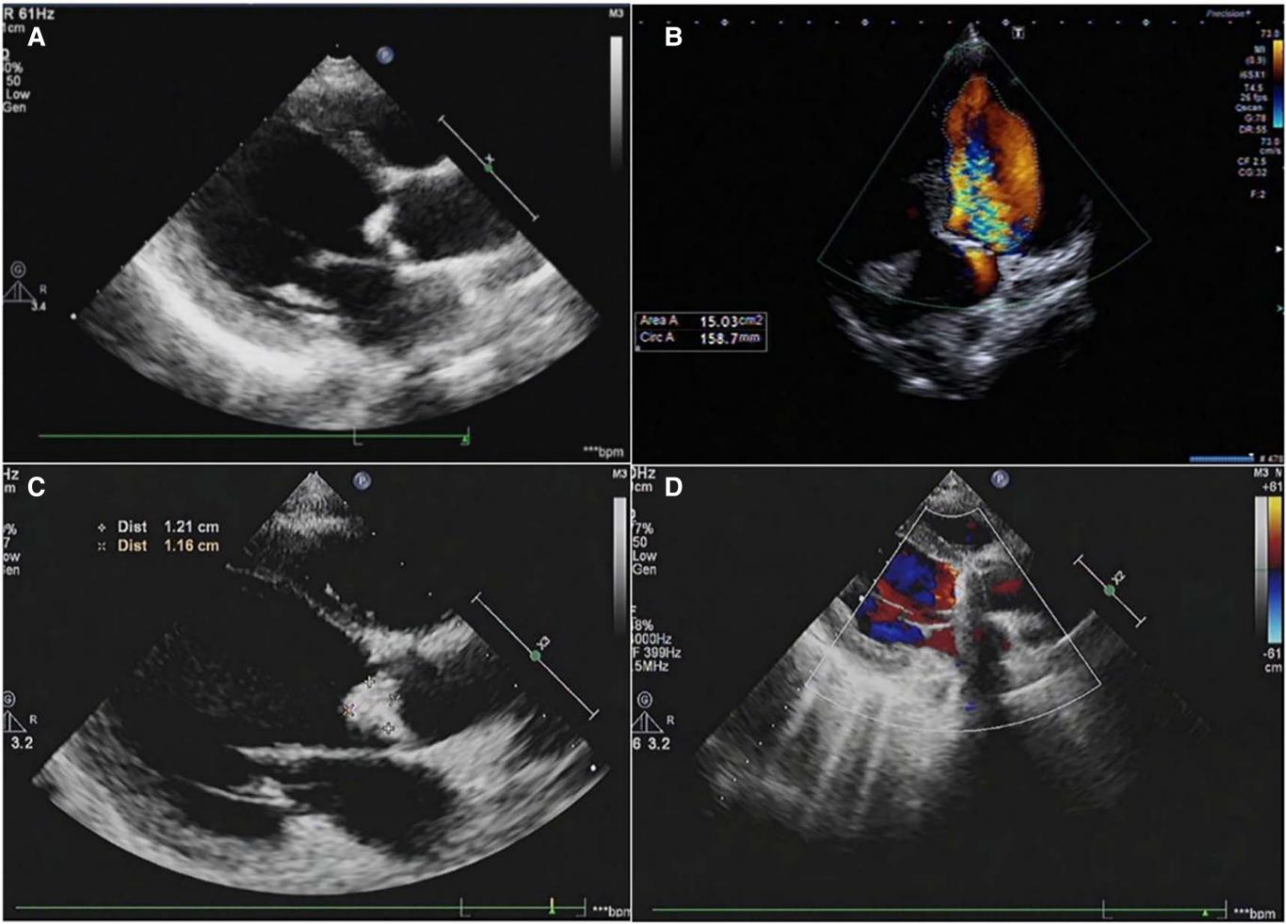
Transthoracic echocardiography findings: (*A*) at admission, demonstrating aortic vegetation; (*B*) at admission, revealing severe aortic regurgitation; (*C*) 1 month post-intracranial haemorrhage, displaying enlarging vegetation; and (*D*) prior to discharge, showing the implanted aortic prosthesis.
